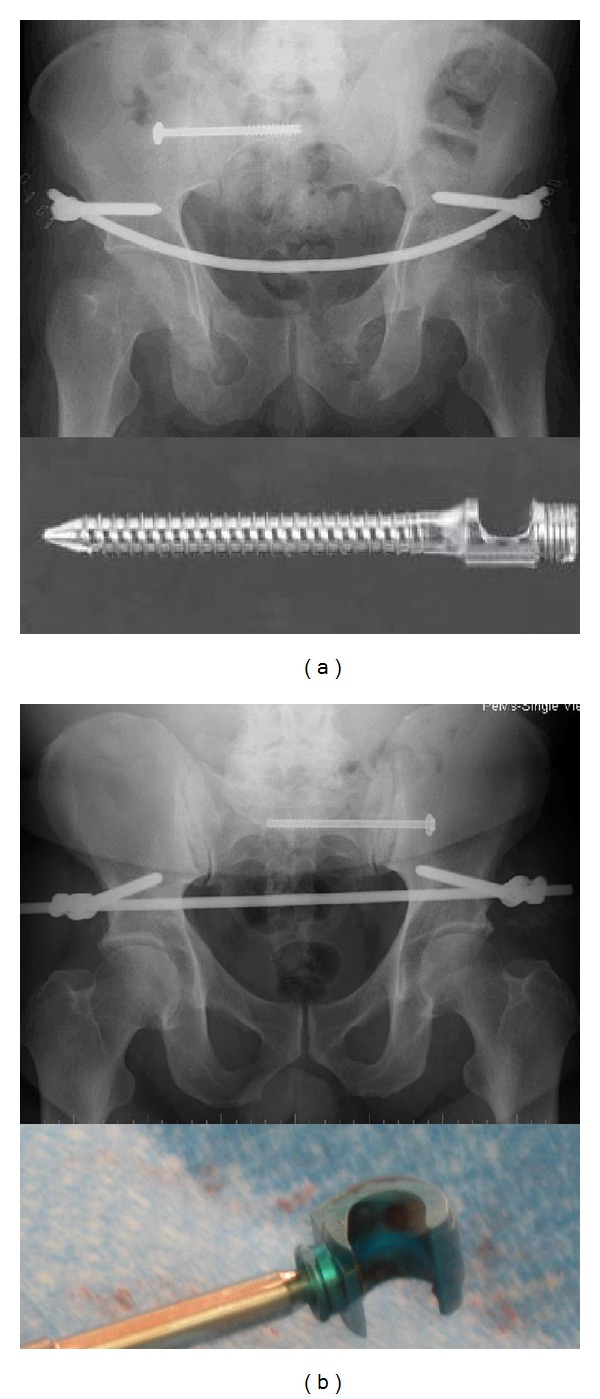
X-rays with an (infix) with (a) a monoaxial system and (b) a polyaxial system reinforced with C-rings.
